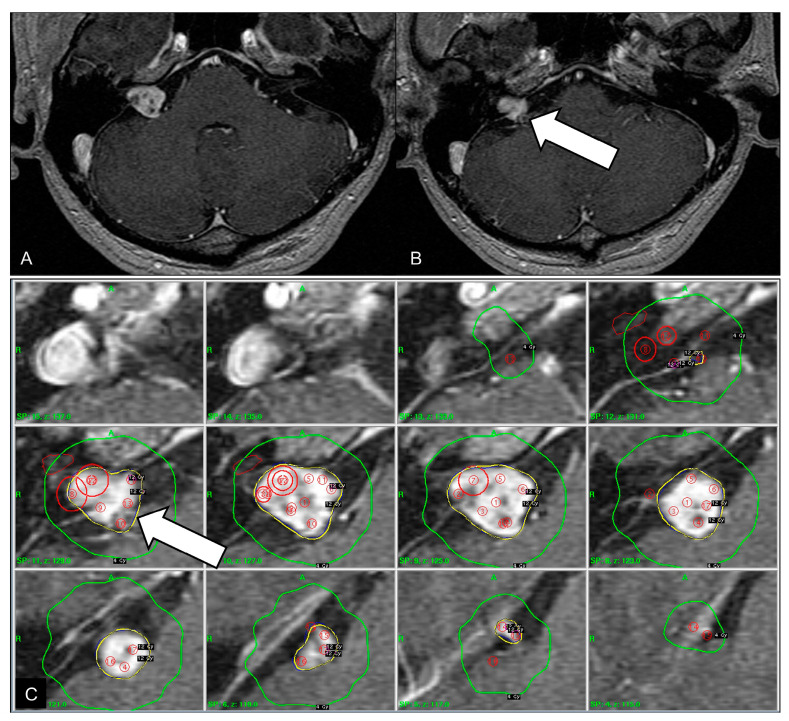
Initial magnetic resonance images before gamma knife surgery. (A) Contrast-enhanced axial T1-weighted image showing a 1.5-cm vestibular schwannoma with internal necrotic content. (B) The inferior part of the vestibular schwannoma is in proximity to the neighboring anterior inferior cerebellar artery (arrow). (C) Axial dose distribution targeting the tumor, based on contrast-enhanced magnetic resonance imaging. The 12 Gy isodense line is outlined in yellow and the 4 Gy isodense line is outlined in green. The 12 Gy isodense curve encompasses the anterior inferior cerebellar artery (arrow).
